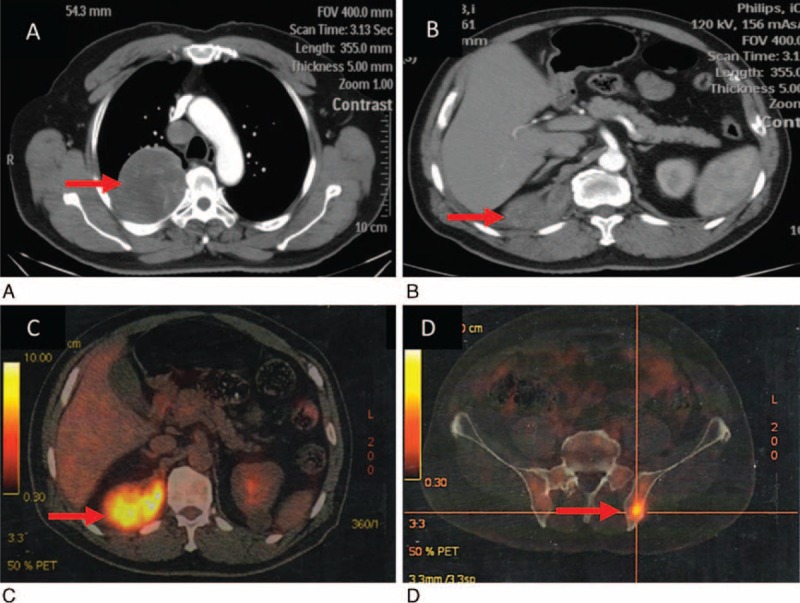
(A) Computed tomography (CT) demonstrated a large right-sided pleural mass with invasion to superior lobe of right lung. (B) CT showed nodule lying in the costophrenic angle. (C) Fluorodeoxyglucose (^18^F-FDG) position-emission tomography (PET)-CT after surgery revealed the residual disease and metastasis of bone (D).
